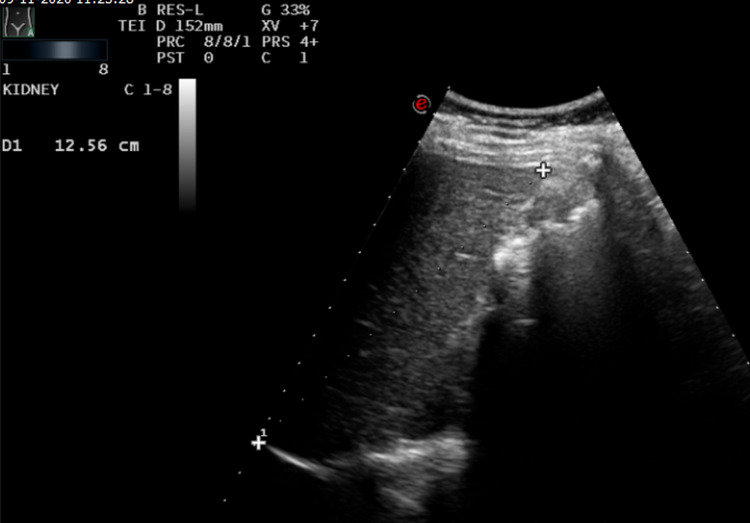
Ultrasound image of the gallbladder fossa showing linear echogenicity representing bowel shadows.
